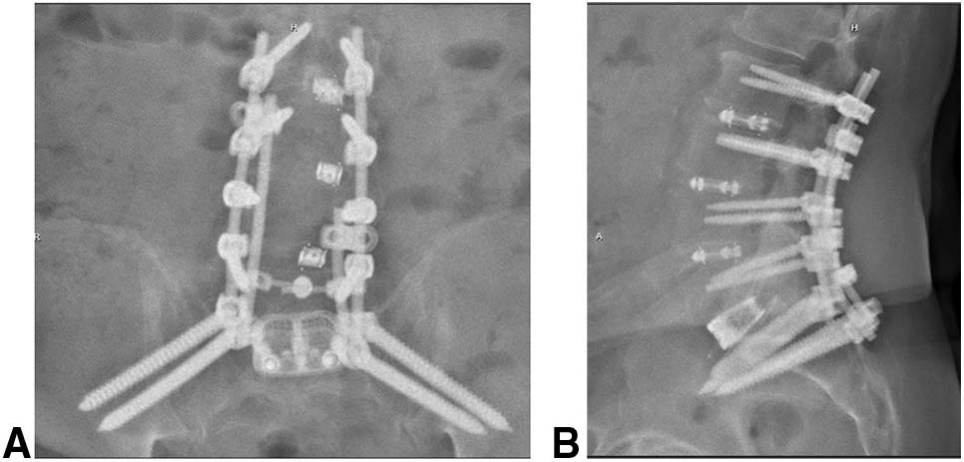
A, Postoperative AP and (B) lateral radiographs of the lumbar spine showing revision of L5-S1 transforaminal lumbar interbody fusion to anterior lumbar interbody fusion with reduction of spondylolisthesis at that level, and reduction of spondylolisthesis and restoration of foraminal height at L5-S1. There is additional pelvic fixation in the form of bilateral sacral-2-alar-iliac screws.
